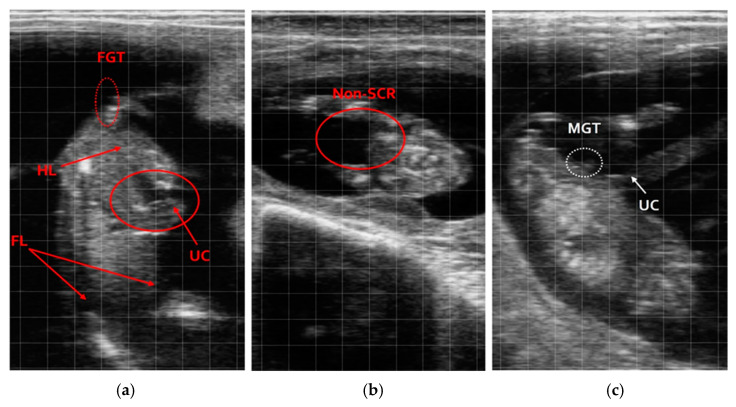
Still image ultrasound of a fetus between 71 and 85 days of pregnancy. (a) Female aerial view; (b) female posterior view; (c) male, hind limbs (HLs); fore limbs (FLs); umbilical cord (UC); male genital tubercle (MGT); female genital tubercle (FGT); scrotum (SCR).
